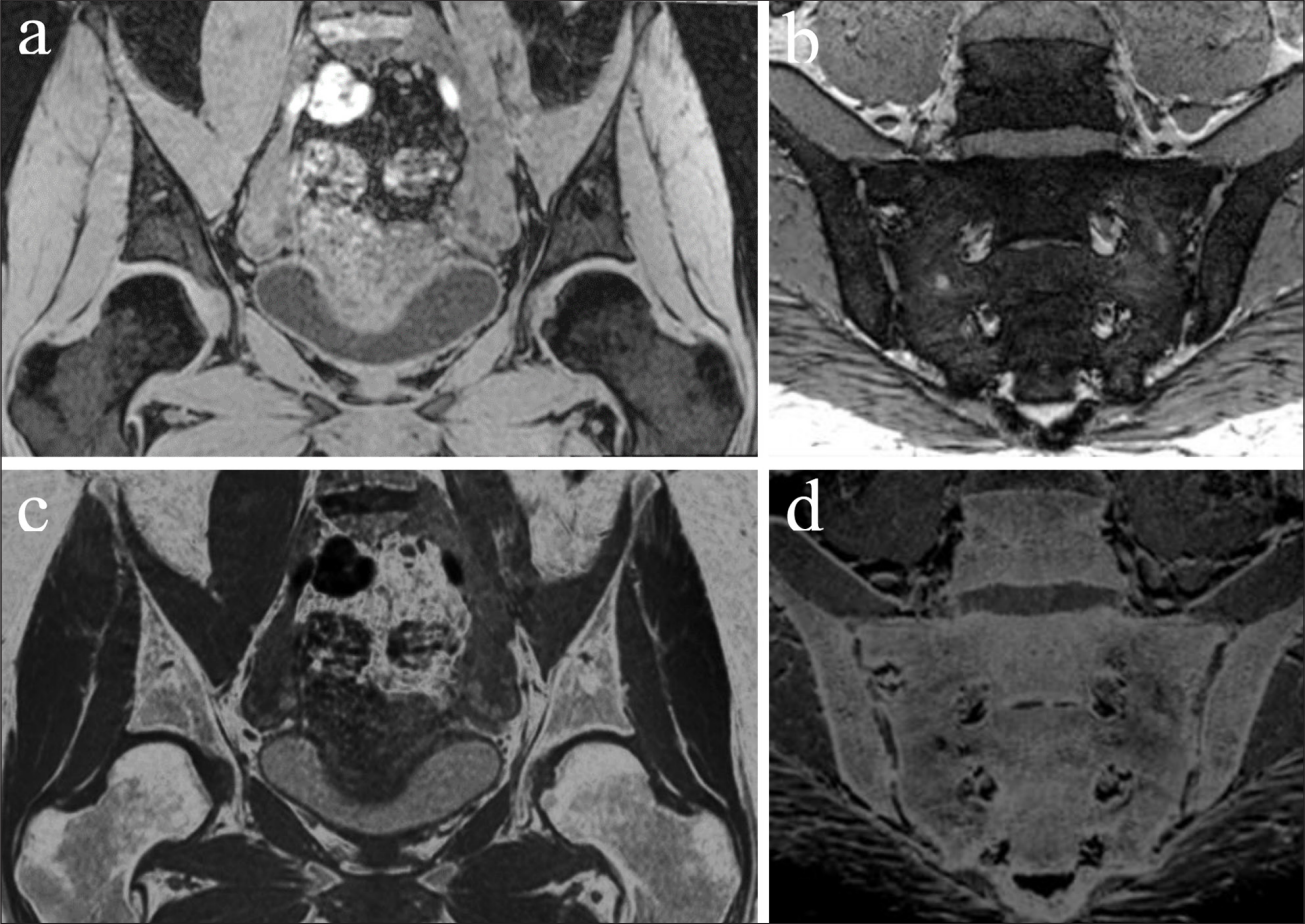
Gradient echo images and susceptibility weighted images (SWI) (a, b) and with inverted grey-scale (c, d). (a) Coronal 3D T1-weighted (T1W) spoiled gradient echo image of the femoroacetabular joints. (b) Semi-coronal SWI magnitude image of the sacroiliac joints. (c) Inverted grey-scale applied on image (a) creating a ‘CT-like’ image. (d) Inverted grey-scale applied on image (b) creating a ‘CT-like’ image.
